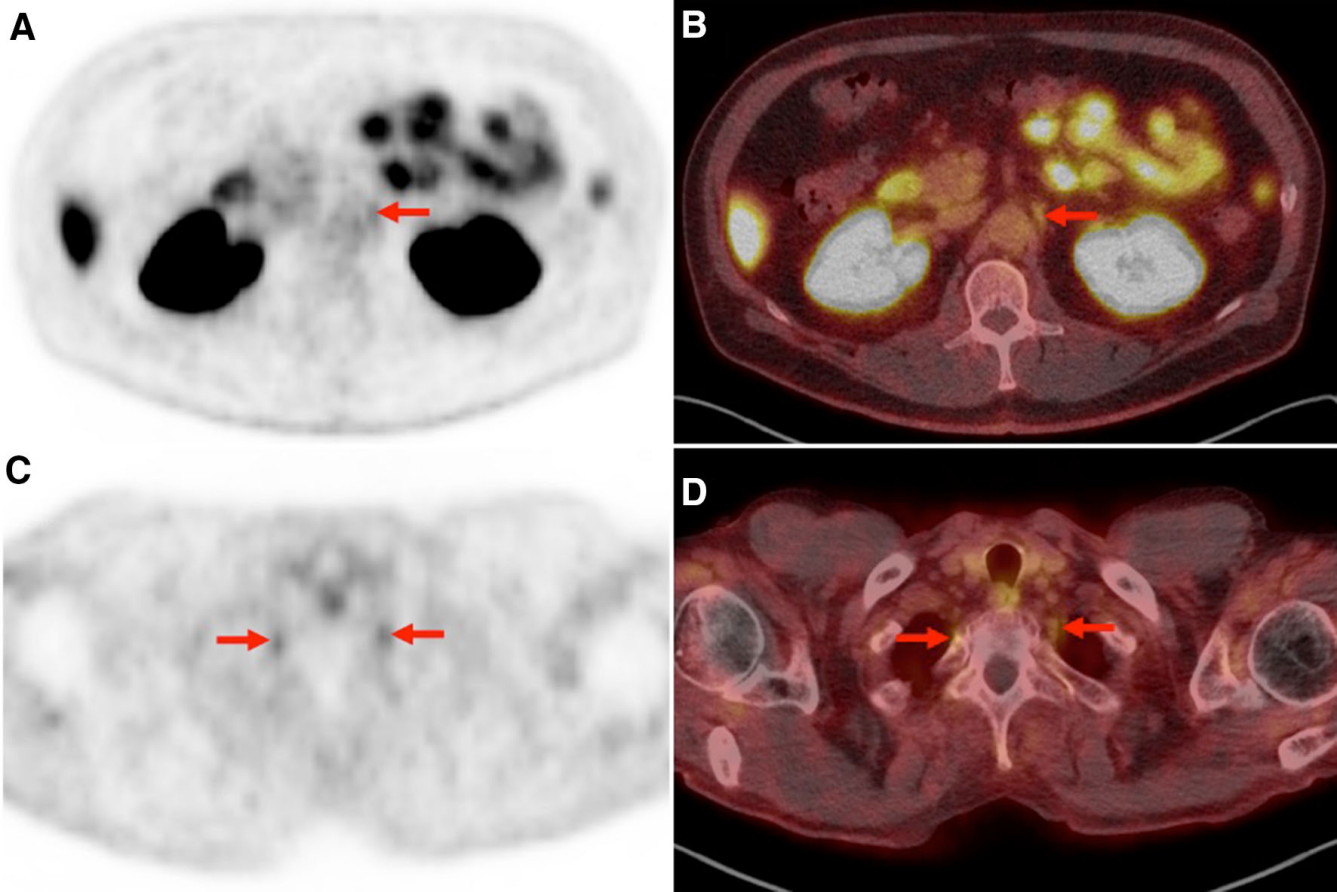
a Axial ^18^F-DCFPyL PET and b axial ^18^F-DCFPyL PET/CT images from a 66-year-old male patient undergoing staging evaluation for recurrent PCa. Focal mild-moderate radiotracer uptake is noted in the left celiac ganglion (*red arrow*). A similar degree of uptake was seen in the right celiac ganglion (not shown). c Axial ^18^F-DCFPyL PET and d axial ^18^F-DCFPyL PET/CT images from a 67-year-old male with recurrent PCa. Mild uptake is present in the stellate ganglia bilaterally (*red arrows*)
